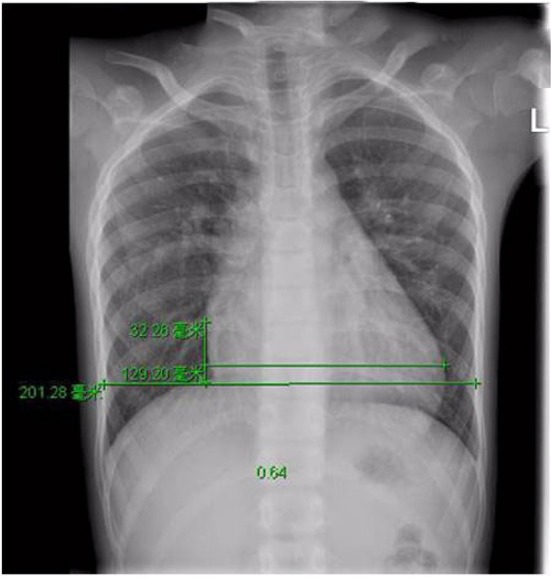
Pre-operative Chest X-ray film view showed increased pulmonary flow and cardiomegaly, with a cardiothoracic area ratio of 0.64.
